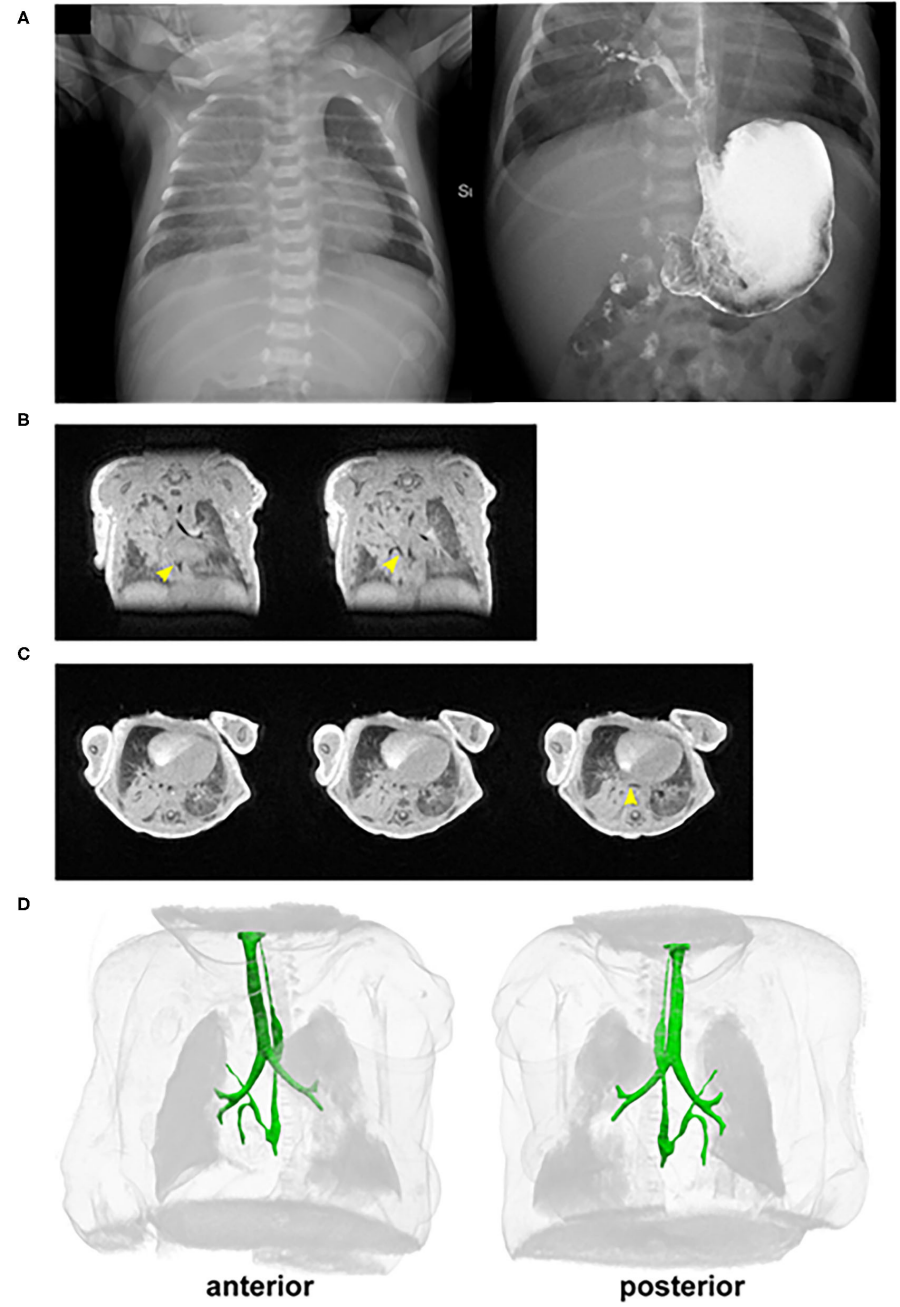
In vivo* imaging of the patient with an esophageal bronchus. (A) Pre-repair/resection of (left) chest X-ray and (right) esophageal contrast study reveals opacification of the right upper lobe and the presence of an esophageal bronchus. (B,C) Selected (B) coronal and transverse (C) cross-sections from UTE MRI. Yellow arrowheads point to the fistula connecting from the esophagus to the right upper lobe of the lung. (D) 3D rendering of the chest from UTE MRI. The foregut (esophagus, trachea, and bronchi) is highlighted in green. The esophageal bronchus is seen with a superior branch proceeding to the right upper lobe. There is also a caudal branch inferior to the crossing of the right main stem pulmonary artery that is narrowed shortly after branching. The narrowing does not appear to be a result of compression by the right main stem pulmonary artery and is presumed to most likely be an intrinsic stricture.
